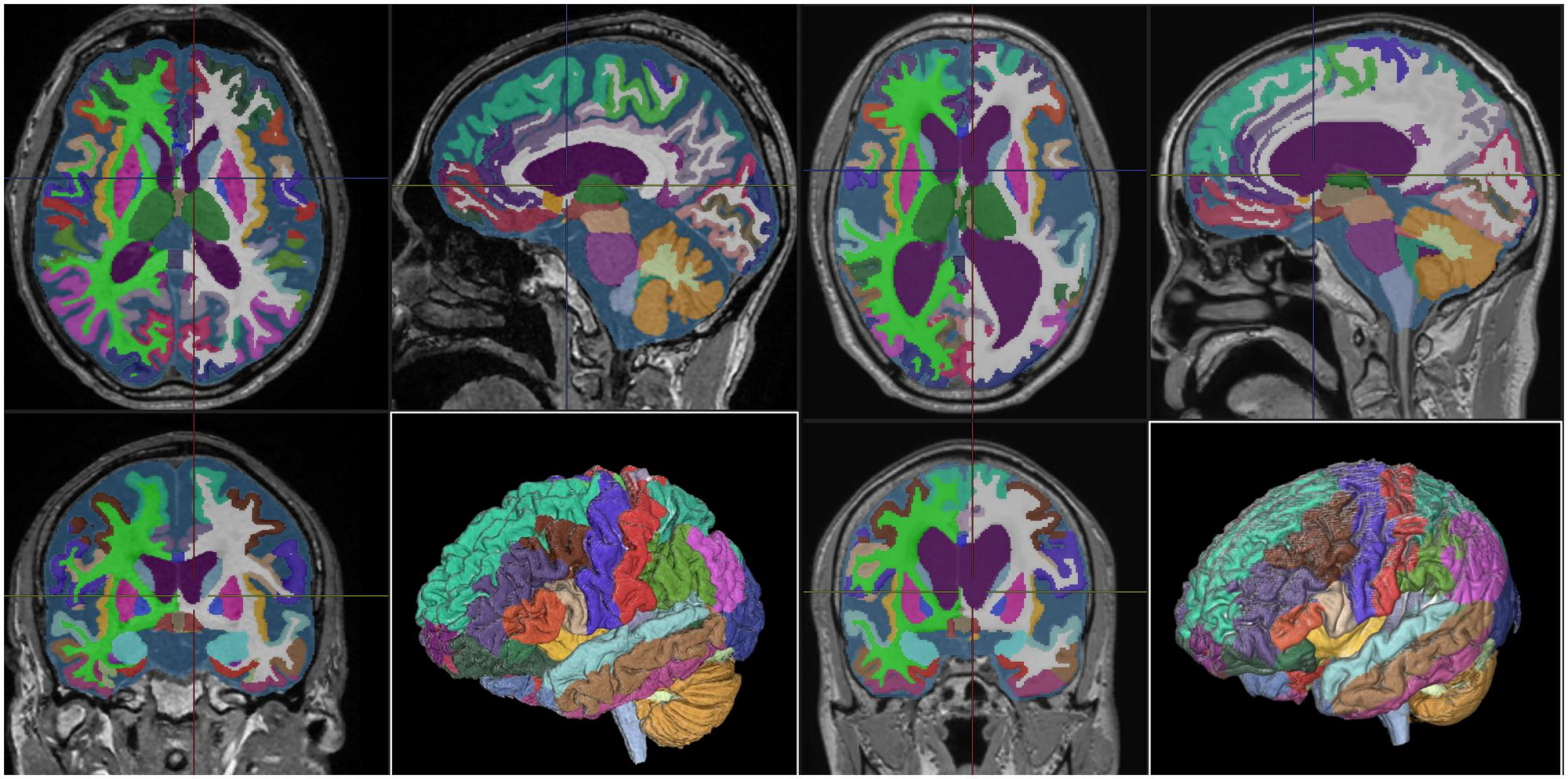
Brain and cerebrospinal fluid (CSF) segmentation from three-dimensional (3D) T1-weighted MRI. The brain subregion analysis application on the 3D volume analyzer SYNAPSE 3D workstation (FUJIFILM Corporation) quickly and automatically segmented the intracranial space into the 100 brain subregions, 4 ventricles, and 1 subarachnoid space from the 3D T1-weighted MRI images. The upper figures show the brain of an elderly healthy volunteer with brain atrophy, whereas the lower figures show the brain of a patient with Hakim’s disease (idiopathic normal pressure hydrocephalus) with a typical disproportionately enlarged subarachnoid space hydrocephalus (DESH) feature.
